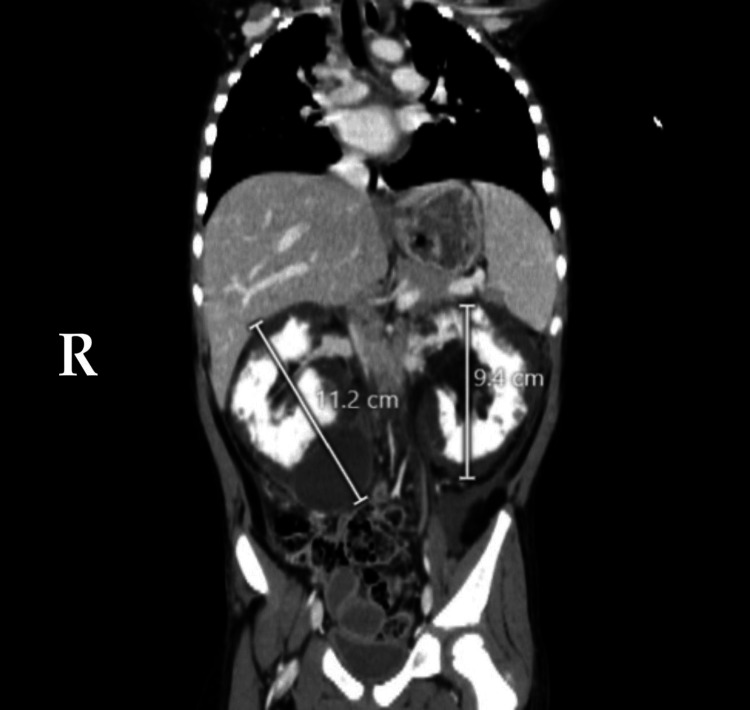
Contrast-enhanced CT of the abdomen (coronal view). Large fluid density collections are noted in bilateral perirenal regions, measuring 11.2 cm on the right side and 9.4 cm on the left side in the maximum craniocaudal (CC) dimension
